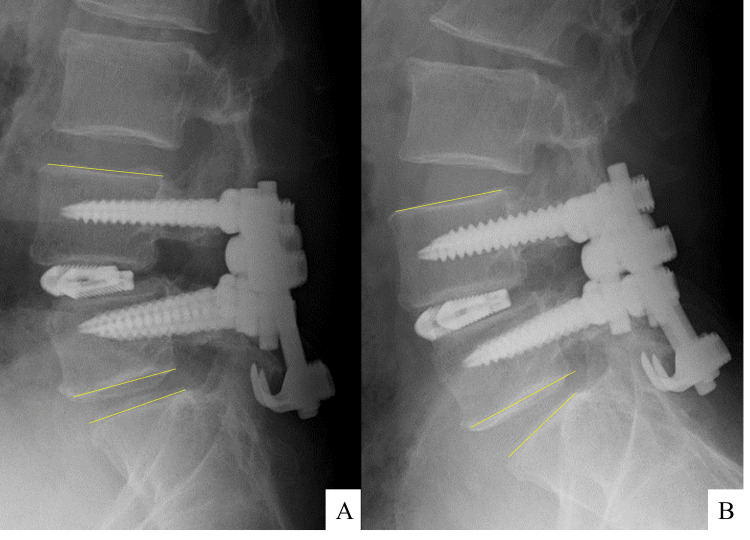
Dynamic X-rays one year after surgery Dynamic radiographs show no angular motion in flexion (A) and extension (B) at L4-L5, with motion preserved at L5-S1 and interbody bony fusion achieved exclusively at L4-L5.
